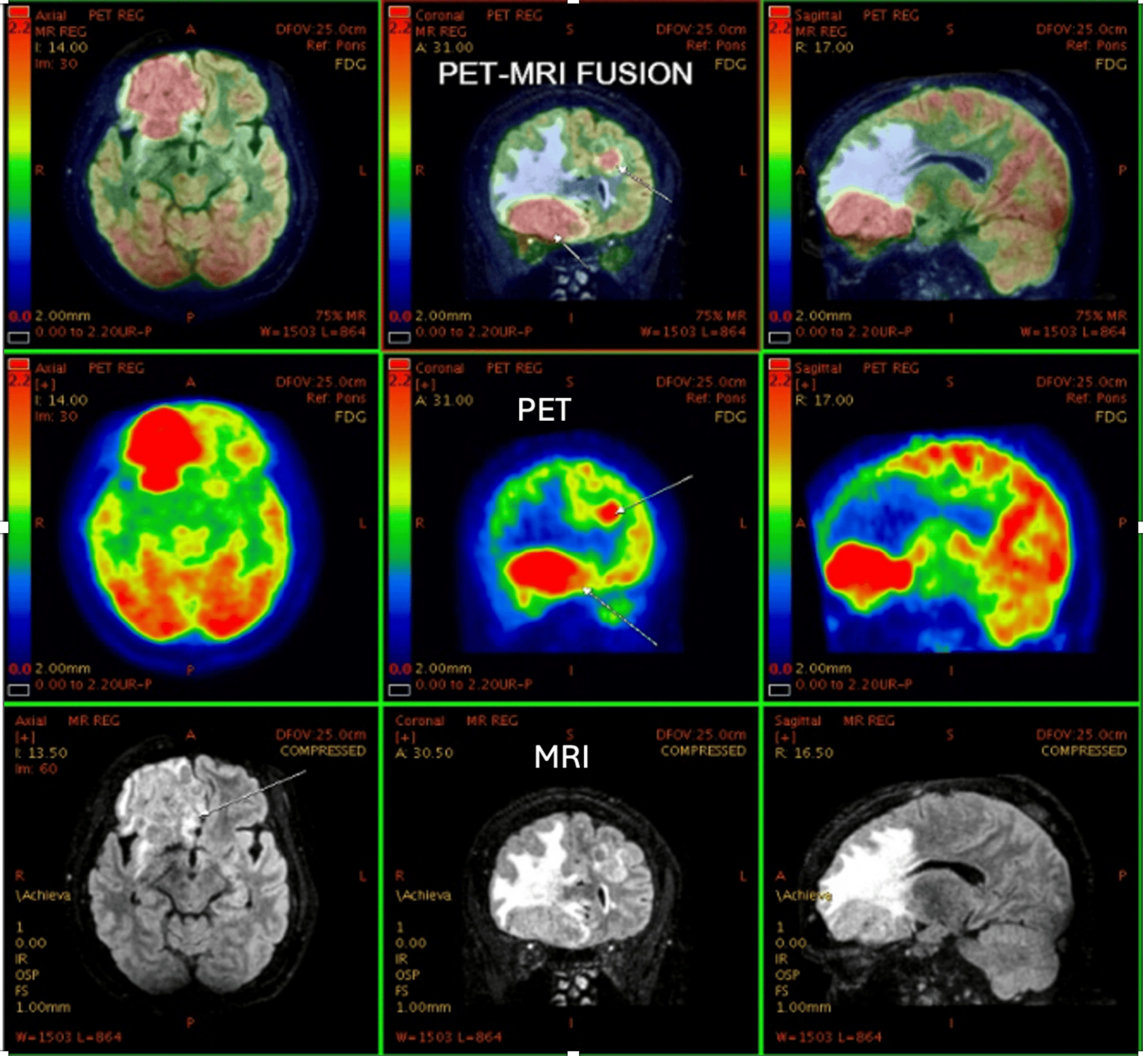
58-year-old female, known case of Ca right breast. Post-operative and chemotherapy status. Presented with complaints of left shoulder pain and lower backache for 15-20 days. CT shows an ill-defined heterogeneous area in the right frontal region. PET-MRI brain study shows heterogeneous large metabolically active lesions in the right basifrontal and left frontal regions with perilesional edema and mass effect, suggesting metastatic lesion. PET: positron emission tomography; MRI: magnetic resonance imaging; CT: computed tomography
